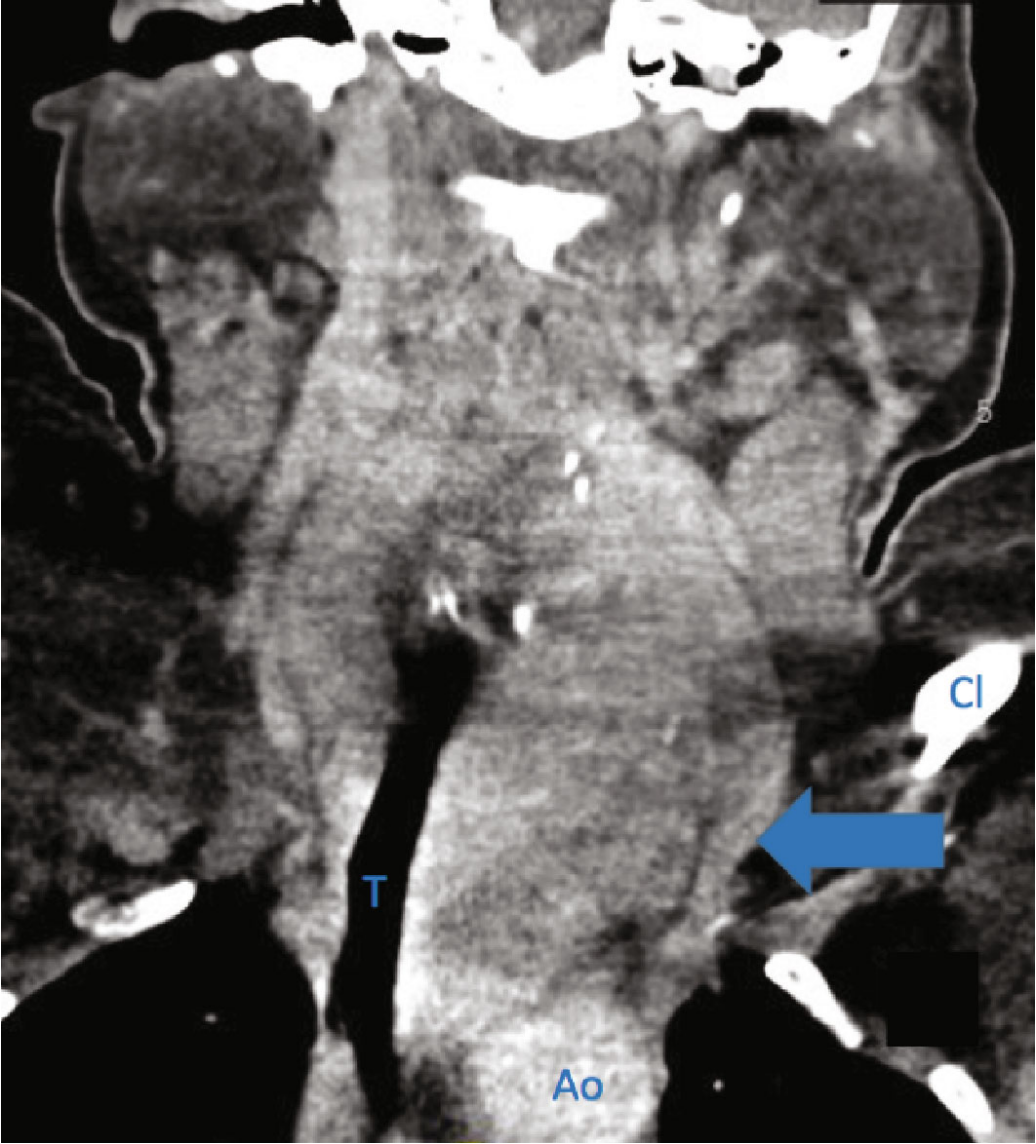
CT neck soft tissue with contrast showing bilateral thyroid goiter with substernal left thyroid extension (blue arrow), rightward tracheal deviation, and mild narrowing. Labels in picture: T: trachea; Ao: aorta; Cl: clavicle.
